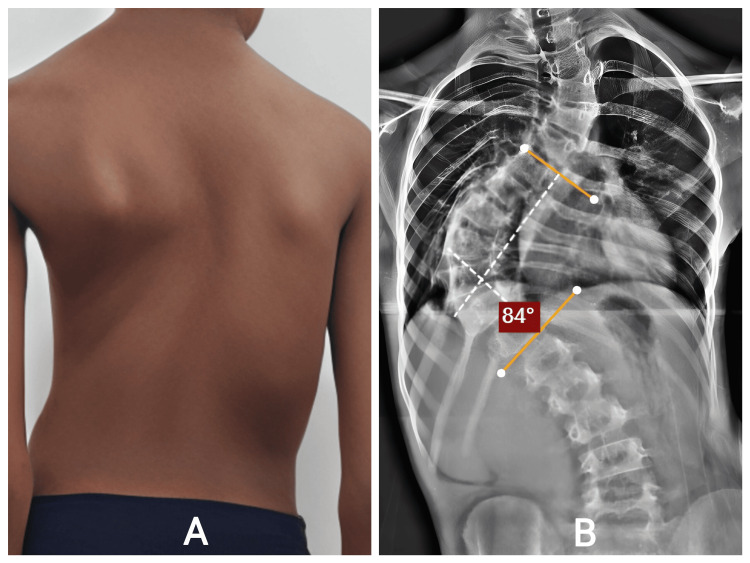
Posterior standing view demonstrating right thoracic curve, shoulder imbalance. B: AP radiograph shows right thoracic scoliosis with dystrophic features typical of NF-1. NF: neurofibromatosis; AP: anteroposterior.
